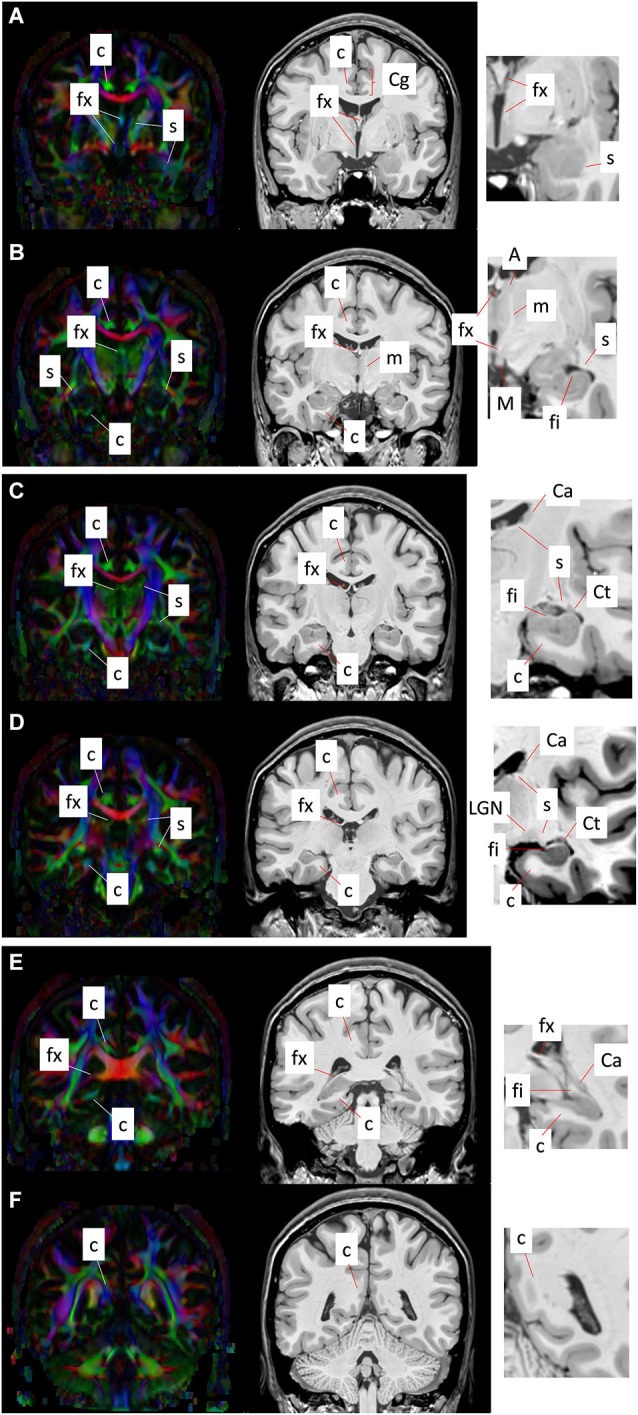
Coronal T1-weighted images (right panel) and corresponding DEC maps derived from DTI (left panel) showing major limbic structures and tracts delineated with MRI contrasts. Images (A–F) show six coronal sections from the anterior to the posterior direction. Red, green, and blue in the DEC maps represent the primary orientation of diffusion along the medial-lateral, anterior-posterior, and superior-inferior axes, respectively. High-magnification views of select regions from T1-weighted contrasts are shown at the right, indicating the gray and white matter limbic structures that can be identified using MRI. Structural abbreviations are; c: cingulum, Ca: caudate, Cg: cingulate gyrus, Ct: tail of the caudate, fi: fimbria, fx: fornix, LGN: lateral geniculate nucleus, m: mammillothalamic tract, M: mammillary body, s: stria terminalis.
